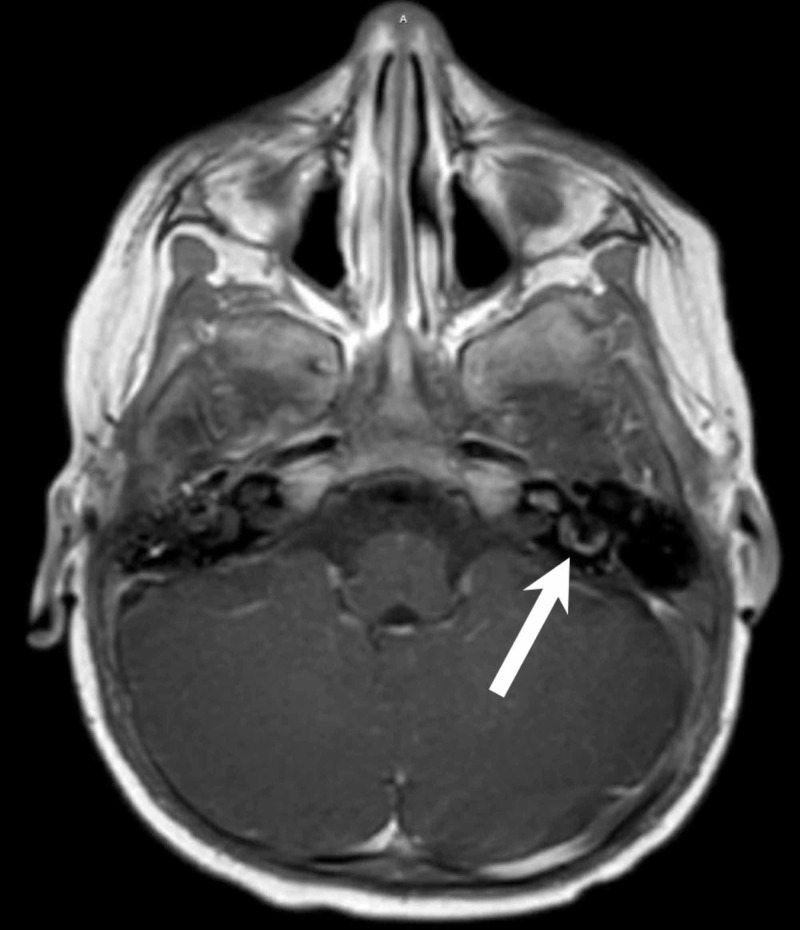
Axial, post-contrast T1-weighted MRI displaying persistent labyrinthitis (arrow).
